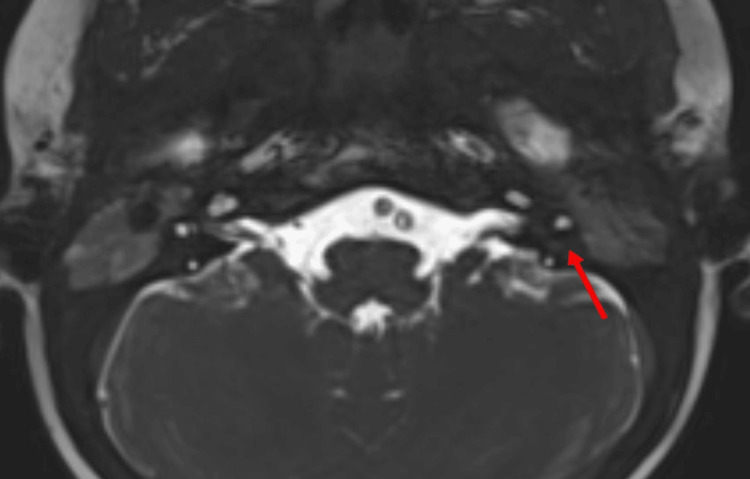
Axial section of the CISS sequence of the MRI of the internal auditory canal It reveals a normal seventh/eighth nerve complex entering the porus acusticus on the right side and the eighth nerve entering the left porus acusticus on the left side. There is aplasia of the seventh nerve on the left side. CISS: constructive interference in steady state
